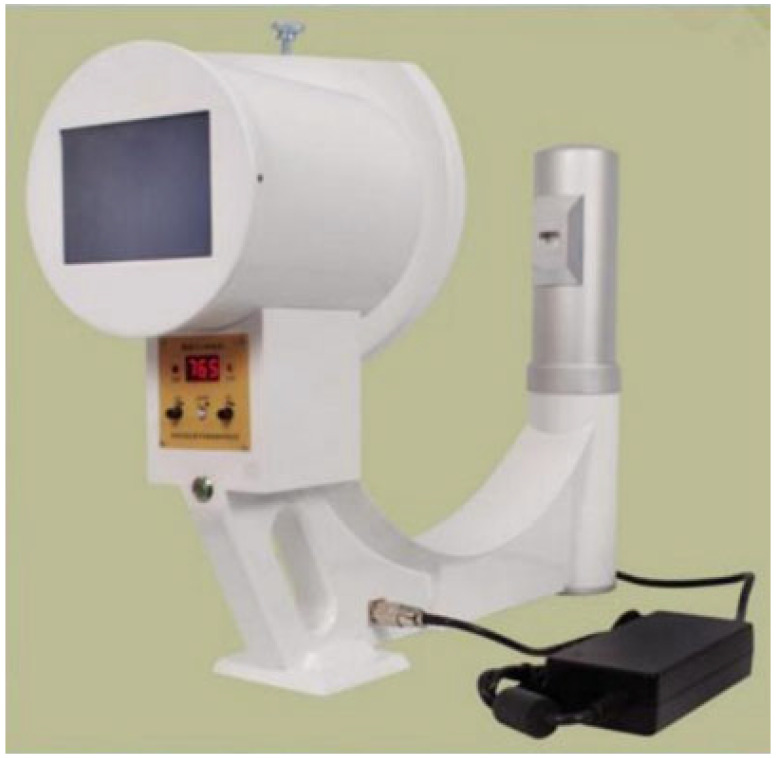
Mobile 1-piece X-ray system.
